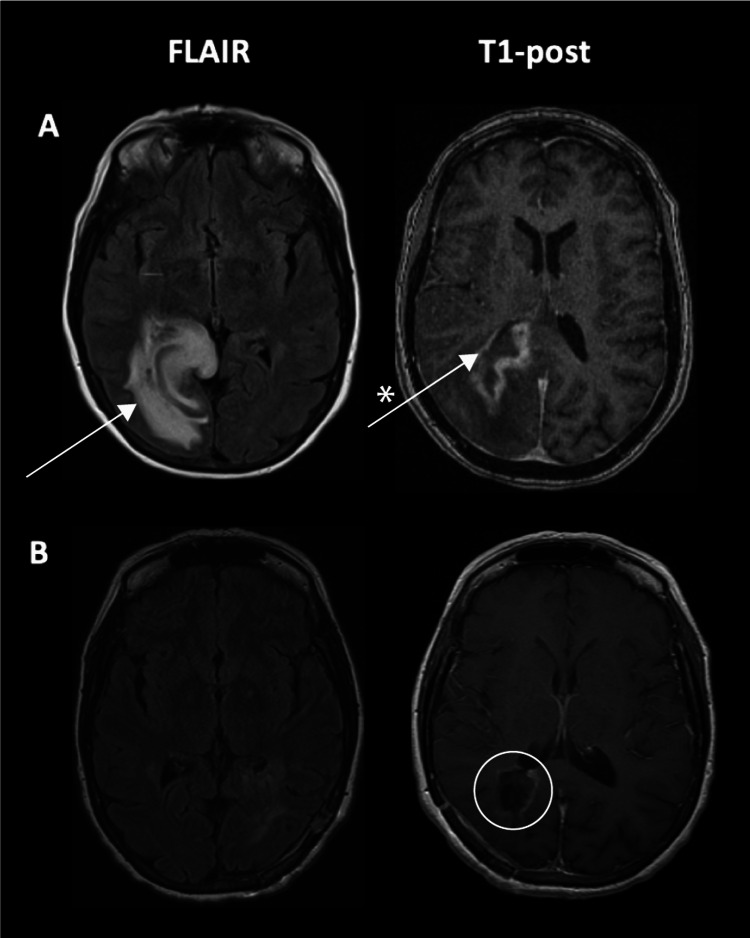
Right parietal mass: Preoperative and postoperative MRI. Two years after symptom onset, the patient developed left homonymous hemianopsia and was found to have a peripherally enhancing and partially hemorrhagic mass centered in the right parietal lobe (Arrow A), extending to the posterior corpus callosum and occipital and temporal lobes, with ependymal and subependymal involvement along the right lateral ventricle (Arrow * A). She was taken for a right parietal craniotomy. Her postoperative MRI showed small residual enhancement along the medial aspect of the resection cavity (Circle B) with near-complete resection of the tumor (B).
